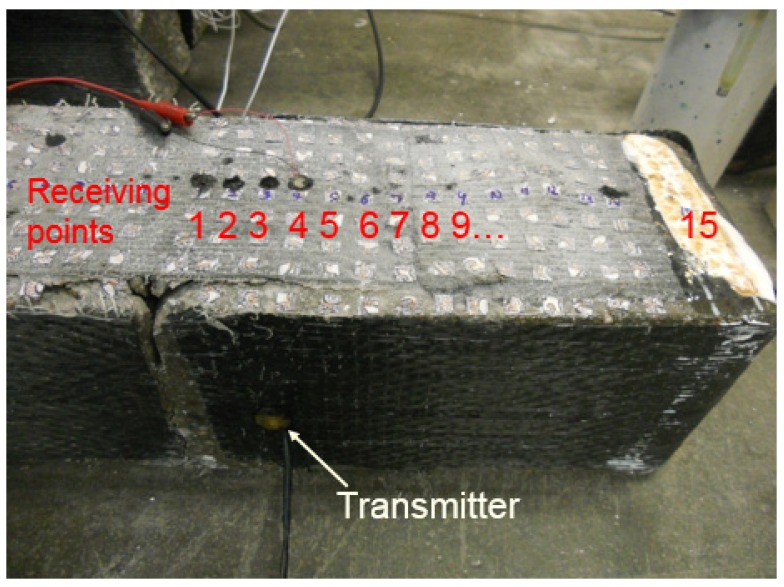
Ultrasonic pitch–catch test setup.
